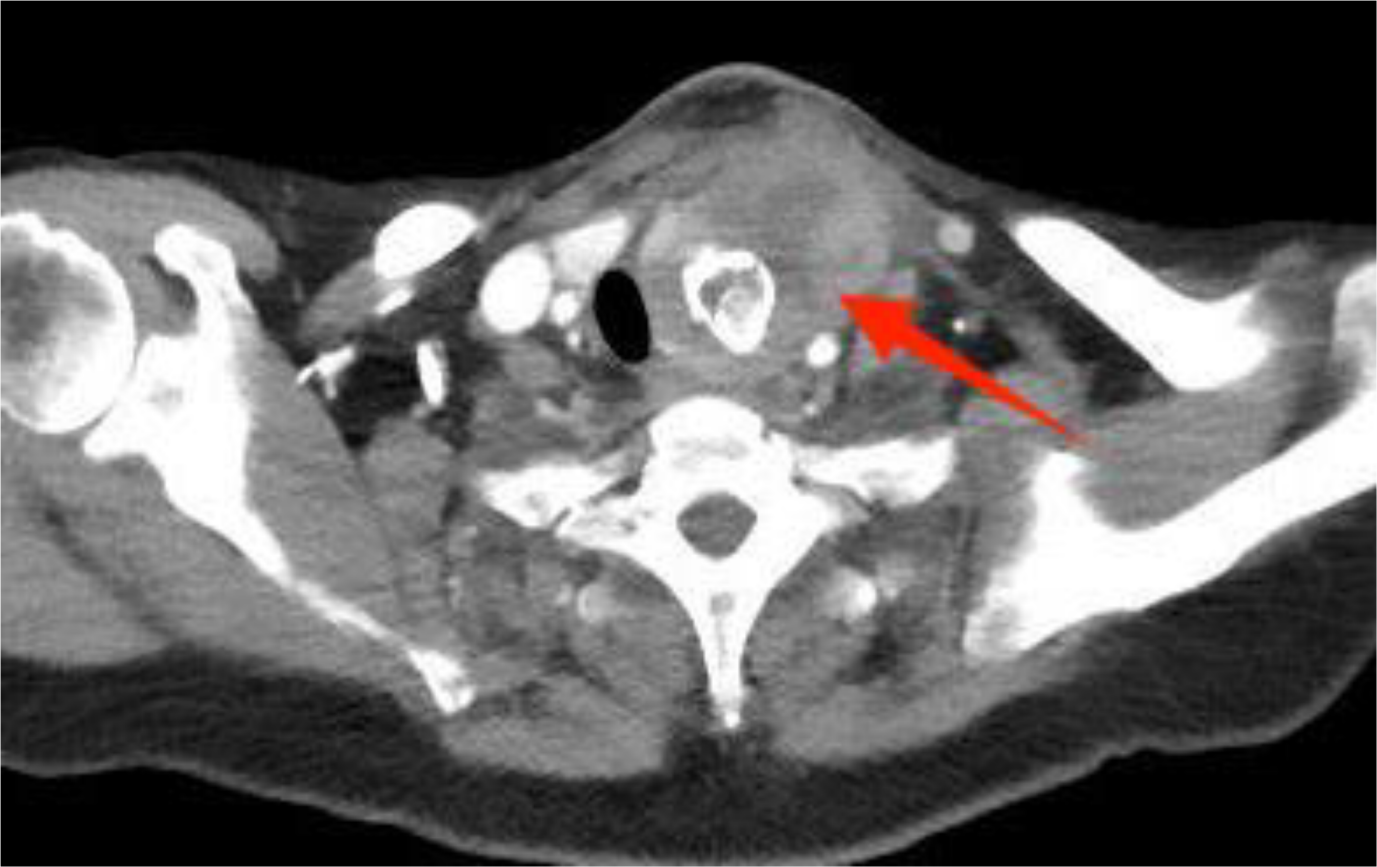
Preoperative contrast-enhanced computed tomography (CT) of the thyroid demonstrated an enlarged left lobe, characterized by internal ring-shaped calcifications and indistinct margins. The trachea was observed to be displaced to the right as a result of compressive forces. Within the mass, areas of low density were identified, suggesting the presence of a lesion, likely indicative of a nodule undergoing liquefaction and necrosis.
